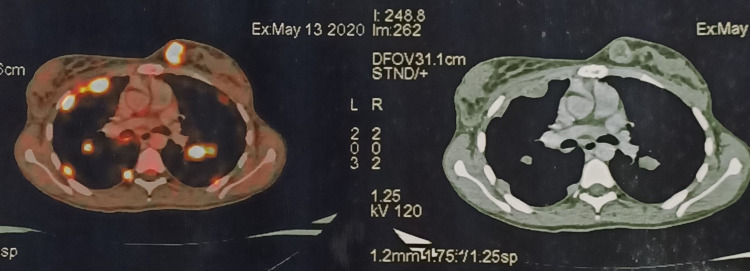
PET-CT scan showing residual disease
